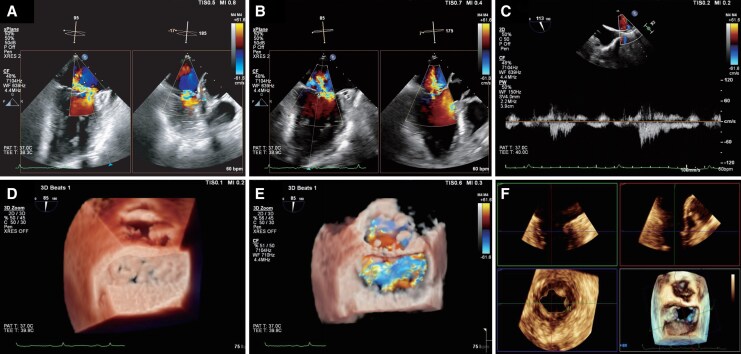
Preprocedural transoesophageal echocardiographic assessment mitral valve (*A*) TEE showing prolapse of the C1 segment and mitral regurgitation. (*B*) TEE showing central mitral regurgitation. (*C*) TEE showing the pulmonary vein flow reversal. (*D*) Three-dimensional TEE showing the location of leaflet prolapse. (*E*) Three-dimensional TEE showing two distinct regurgitant jets. (*F*) Assessment of mitral valve area. TEE, transoesophageal echocardiography.
